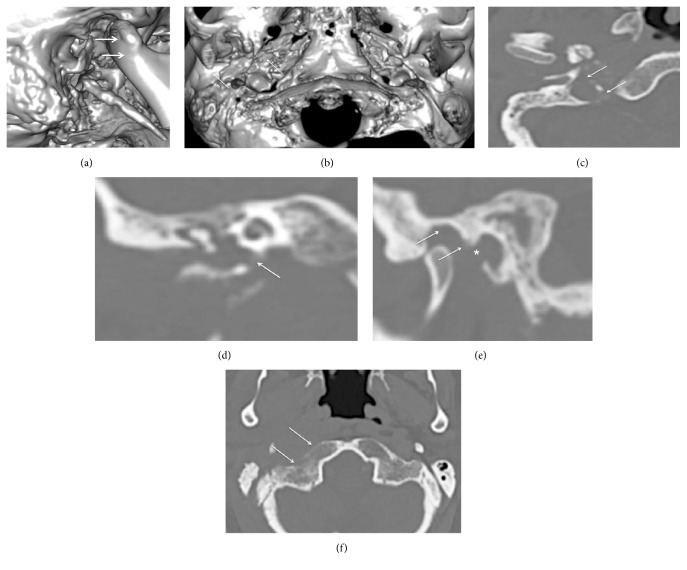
(a) The 3D volume rendering technique shows the lateral surface of the temporal bone: the destruction of the base of the styloid process (vaginal process) is clearly seen. Anterior dislocation of the mandible with subluxation of the condyle is shown with arrows. Note slight cortical erosion of the mastoid process of the right side. (b) Inferior view of the skull base: massive destruction of the petrous and tympanic parts of the temporal bone is marked with arrows. (c) The most severe bone lytic changes were observed around the carotid foramen (arrows). (d) A destruction of the carotid plate is clearly seen on this coronal reformat (arrow). (e) The other possible infection-spreading pathway was from the external auditory canal. Asterisk shows a defect in the anterior wall of the latter. Additionally, arrows show erosion of the mandibular fossa roof as well as auricular tubercle. Note an anterior dislocation of the mandibular condyle. (f) Axial CT scan shows the destruction of the cortical layer of the occipital bone (arrows).
